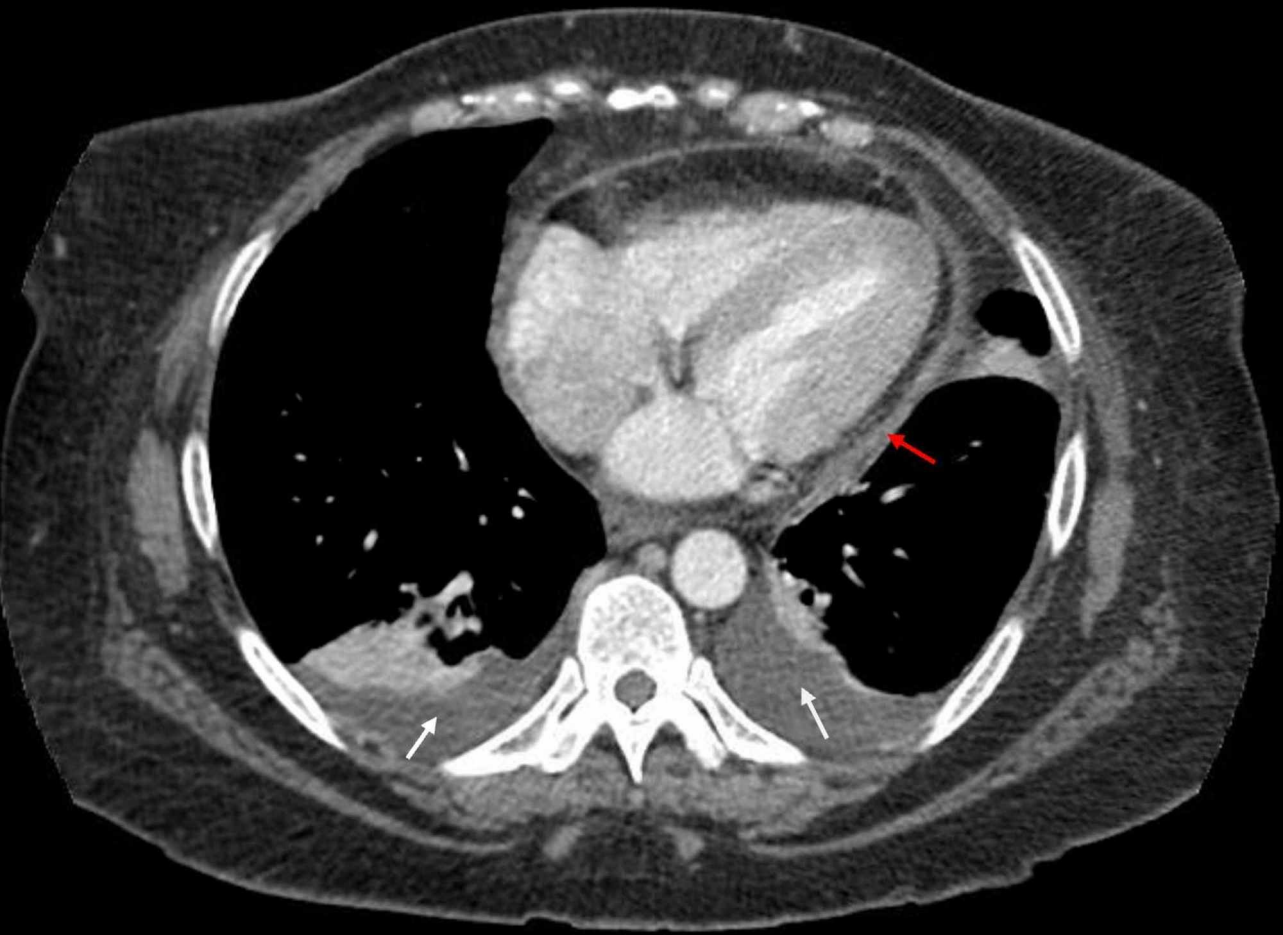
CT thorax showing pericardial effusion (red arrow) and bilateral pleural effusion (white arrow).
